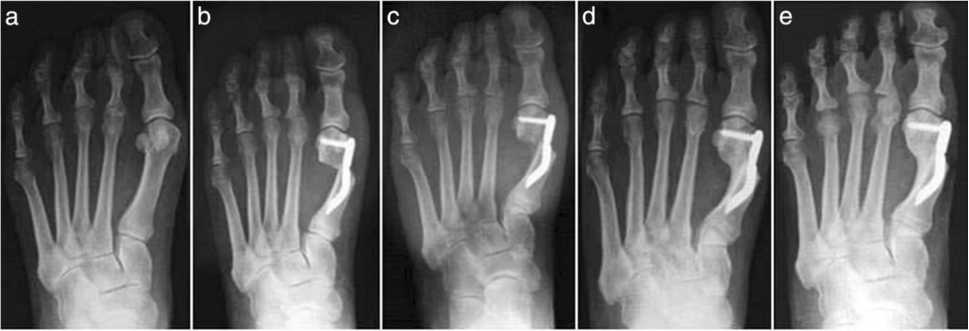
Case 1. A 44-year-old woman with mild hallux valgus (HV). a Preoperative antero-posterior radiographic image. b Endolog translation more than 100 % at 1-month follow-up, X-ray image. c Bone callus formation at 3-month follow-up, X-ray image. d a at 6-month follow-up X-ray image. e Radiographic aspect at 48-month follow-up showing the maintained correction of the deformity and complete healing of the osteotomy
